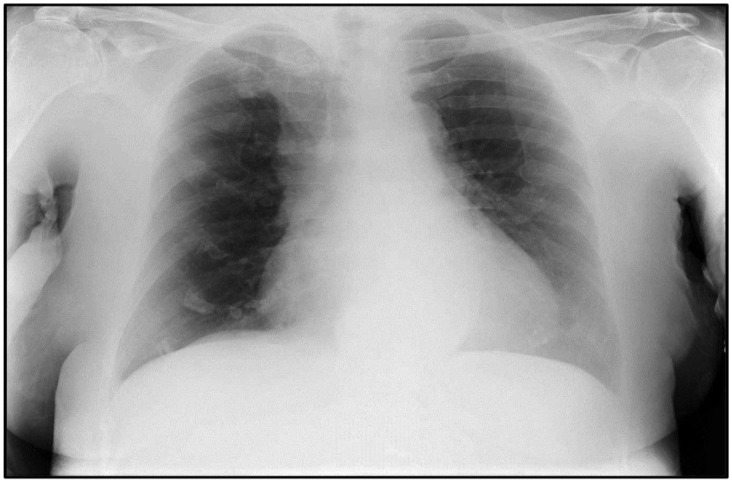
Chest X-ray of case #1 showing left ventricular procidentia with no sign of SARS-CoV-2 pneumonia.
